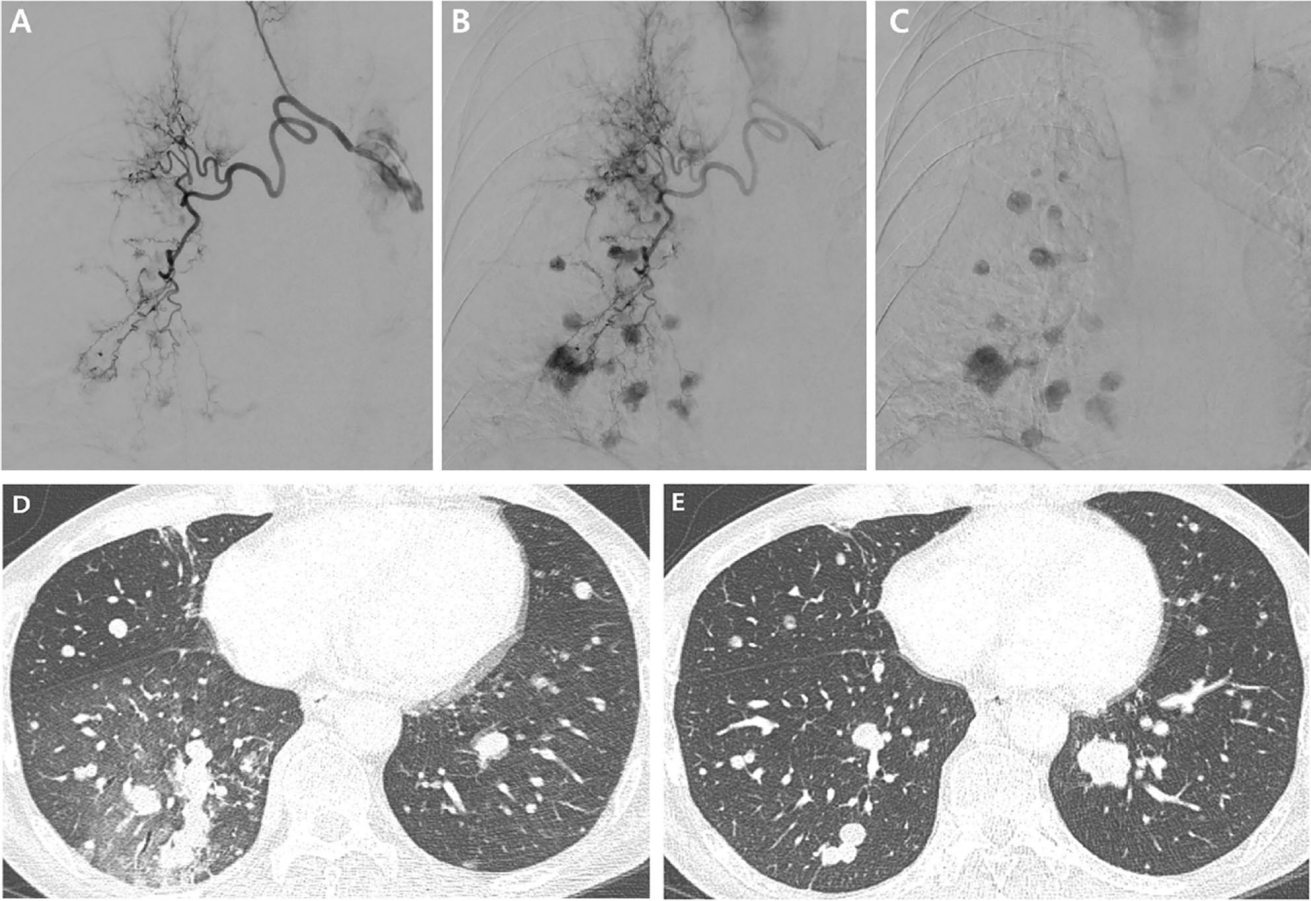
A 57-year-old man with pulmonary metastasis from HCC who presented with a moderate amount of hemoptysis. (A–C) Selective right bronchial arteriography using a 5-F catheter shows a tortuous hypertrophic right bronchial artery and multiple nodular hypervascular tumor blushes. Embolization of the right bronchial artery was successfully performed using hystoacryl (NBCA: lipiodol = 1:10) and the patient showed complete resolution of hemoptysis after bronchial artery embolization. (D) Axial CT image before embolization shows multiple nodular pulmonary metastases in both lungs, particularly prominent in the right lower lobe, with patchy ground glass opacities suggesting aspirated blood. (E) Axial CT image 1 month after embolization of the right bronchial artery shows the metastatic nodules in the right lung to be decreased in size and number, but an aggravated pulmonary metastasis that was not in the embolized territory is visible in the left lung.
